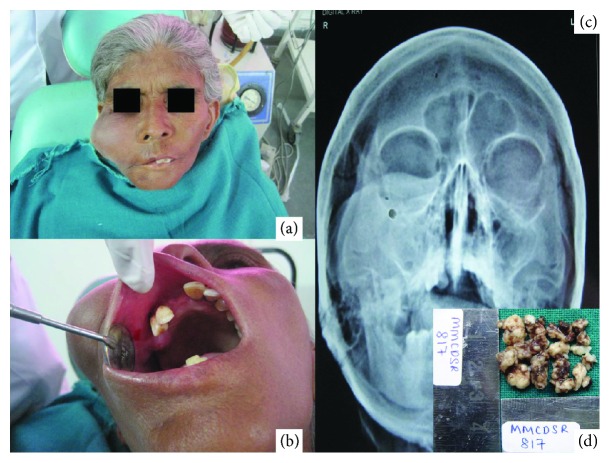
(a) Clinical and (b) intraoral picture showing an intact buccal mucosa. (c) Water's view radiograph of the patient. (d) Gross incisional tissue.
